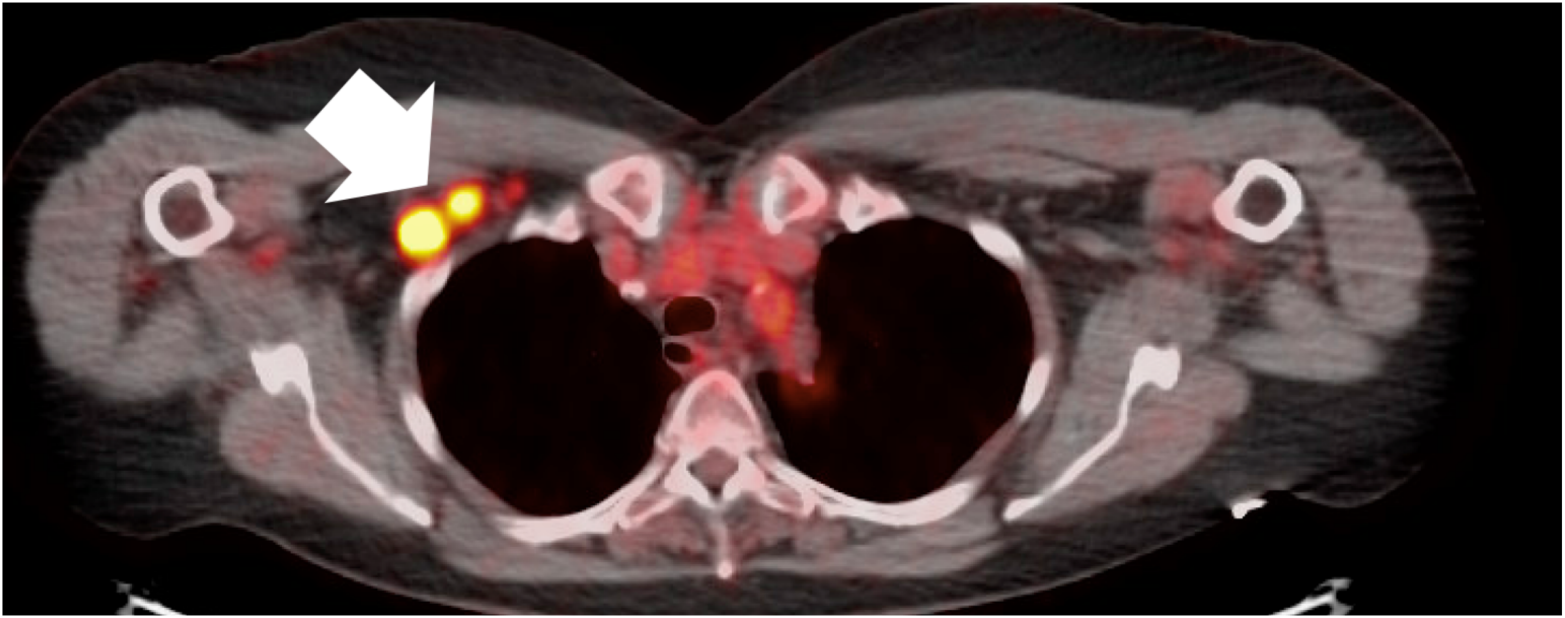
FDG-avid right axillary lymph nodes, biopsy-proven Stage I Hodgkin lymphoma.
